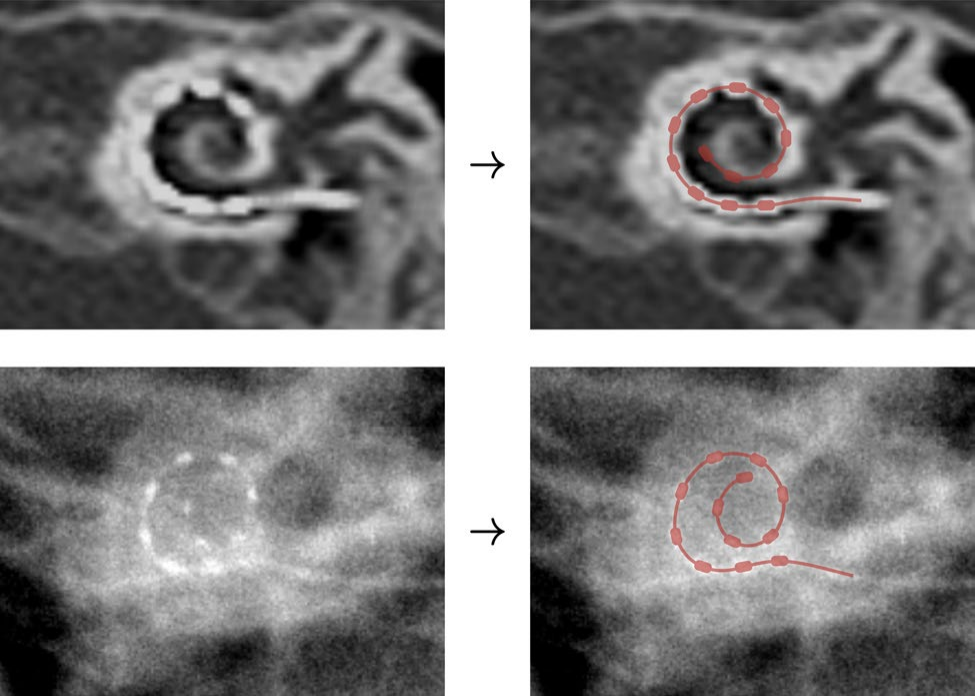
Postoperative CT scan (top) and X-ray (bottom) for the assessment of the electrode array position. The twelve electrode contacts are highlighted in red
